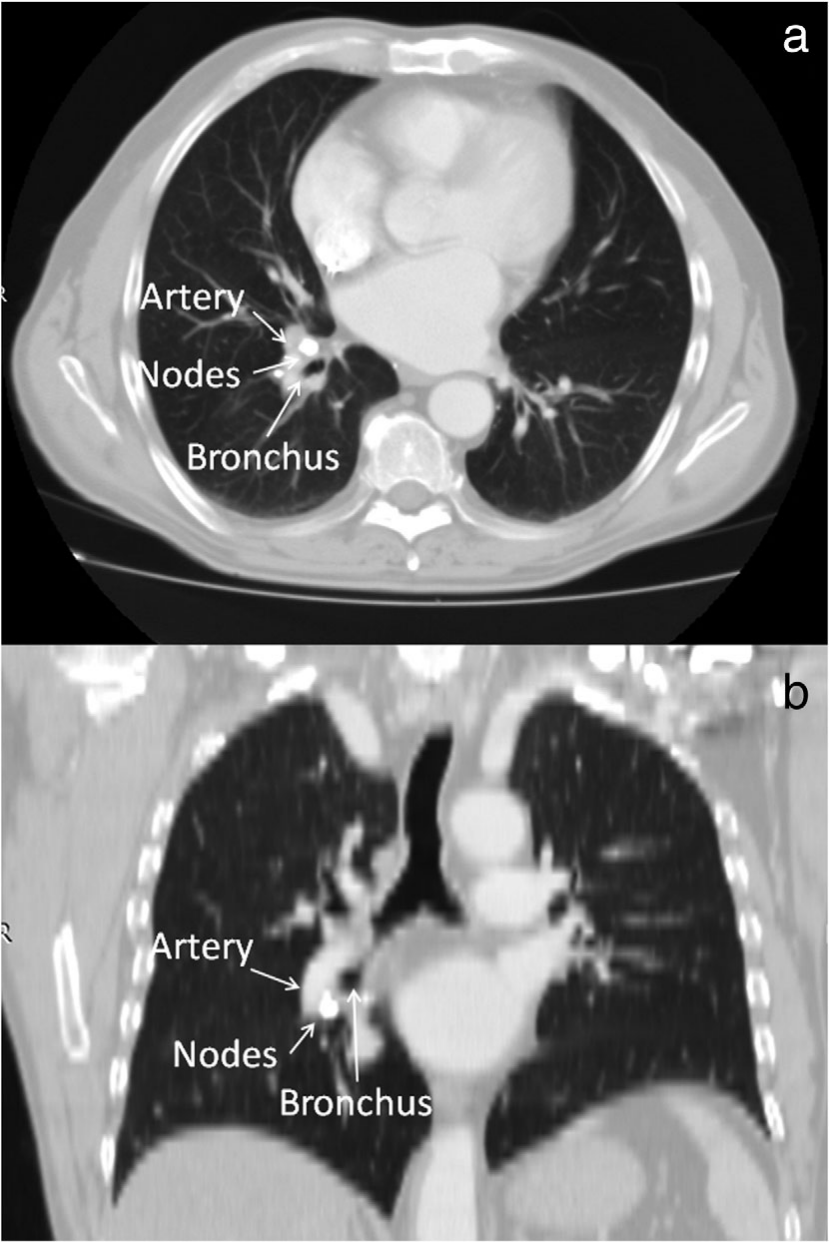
Computed tomography (CT) findings. (a) Axial view, and (b) coronal view showed calcified lymph nodes between lower pulmonary artery and lower bronchus (patient number 3)
